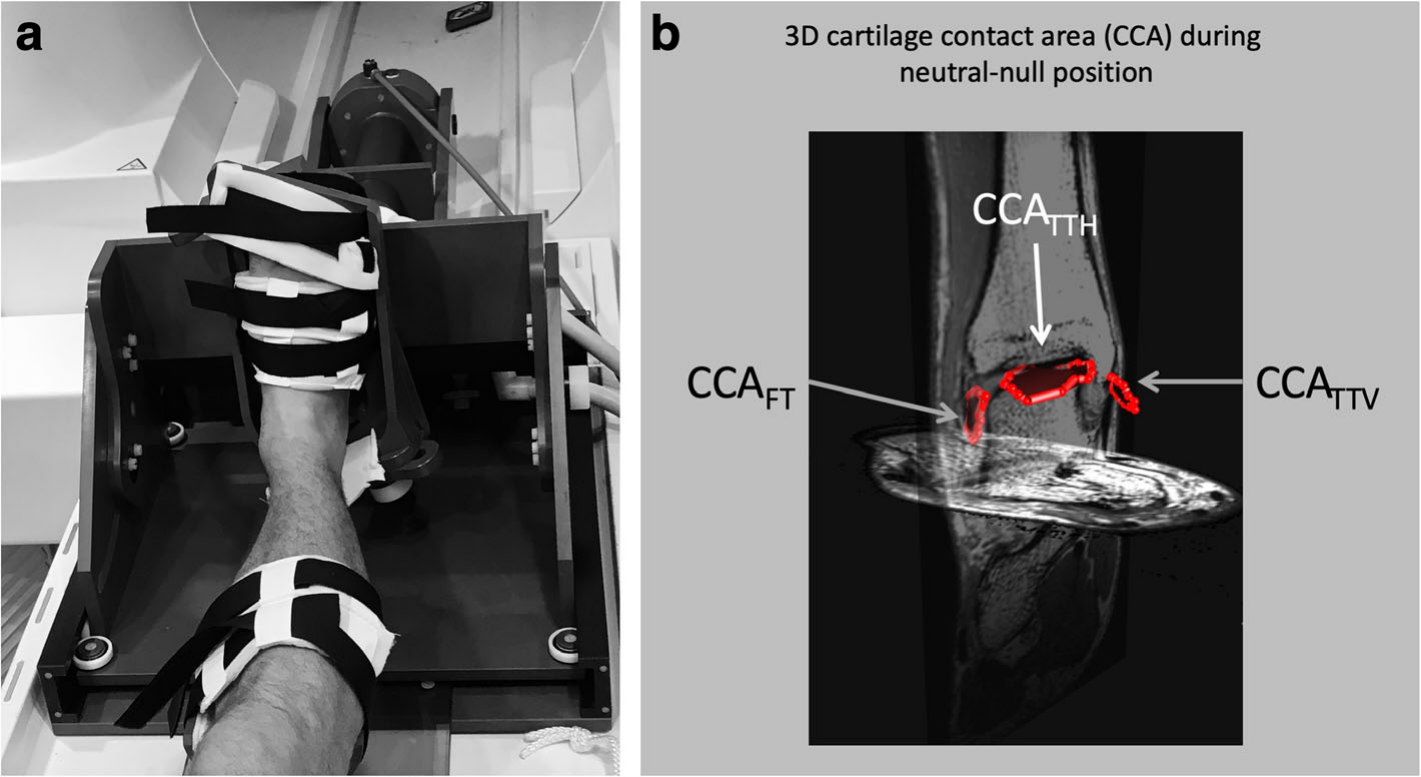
a Foot placed in custom-built MRI arthrometer during 3SAM. b MRI image showing the three different parameters deducted from 3SAM
